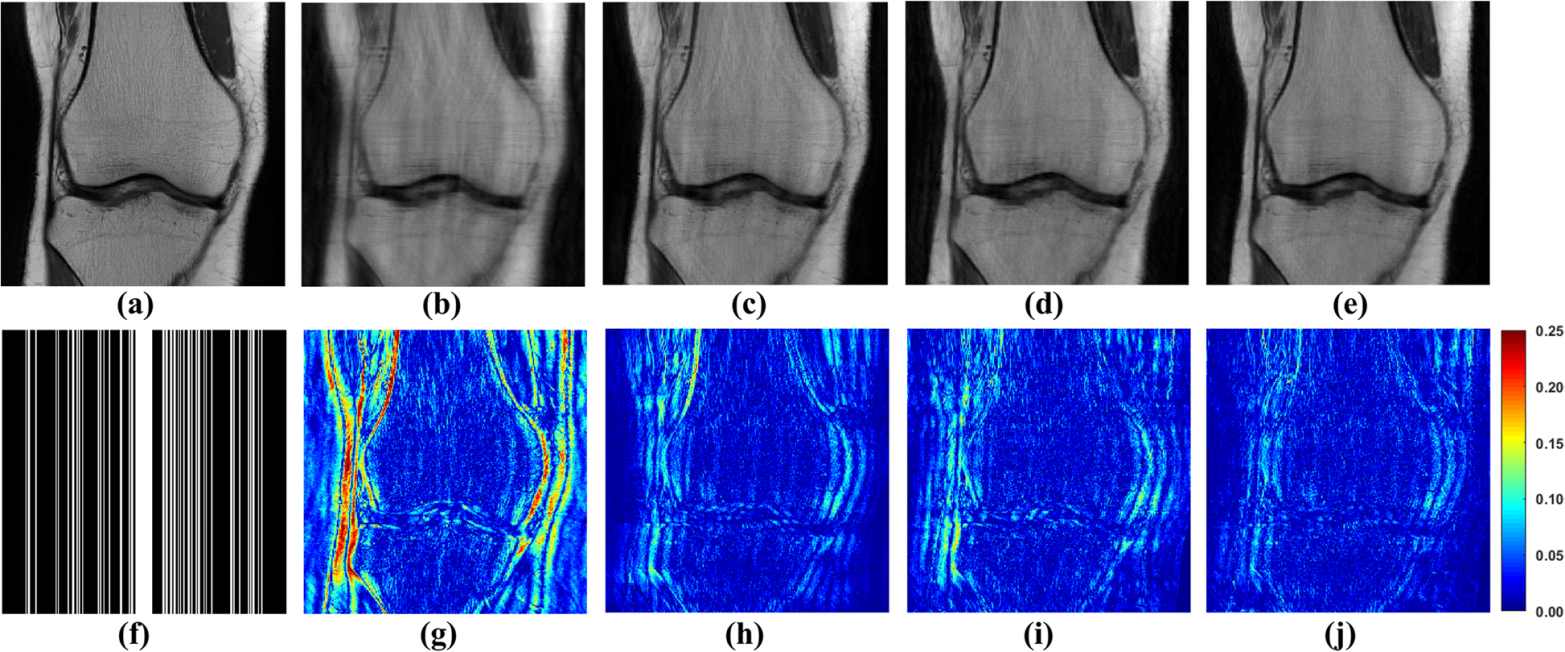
The proposed GCN for MRI reconstructions. a Is the fully sampled image. b Is the undersampled image. f Is the 1D Cartesian undersampling pattern with AF = 4. c-e Are reconstructed images by SPIRiT, UnGCN, and RecGCN, respectively. g-j Are the corresponding error maps
